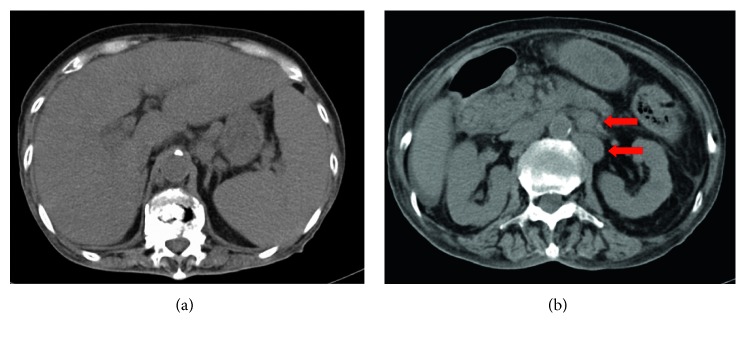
CT image prior to carfilzomib administration. (a) Low uptake throughout the entire liver and splenomegaly could be observed. (b) Para-aortic lymph node swelling was observed (red arrow).
